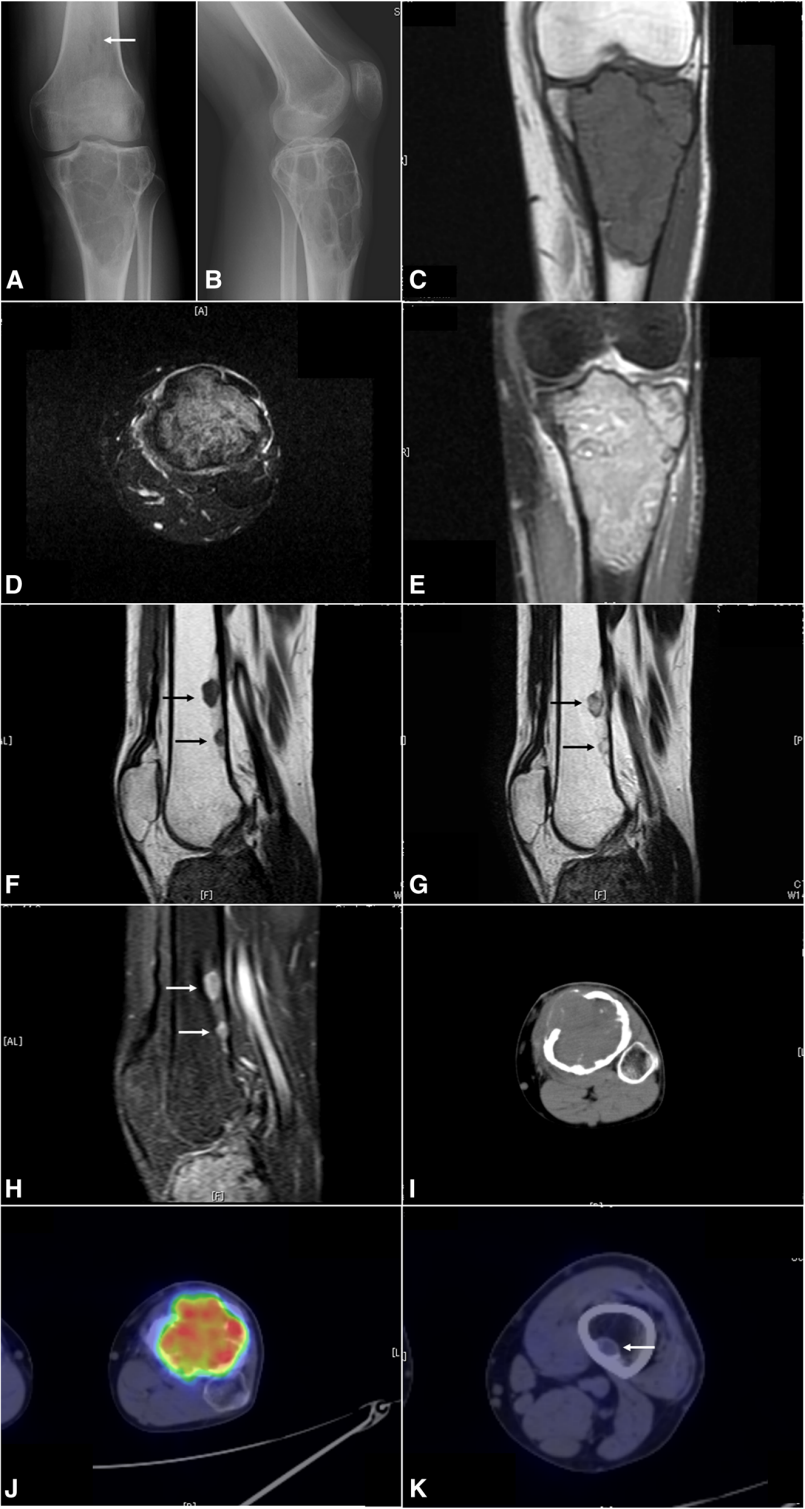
Pre-treatment radiological analyses of the left knee of the patient. Plain radiographs show a soap-bubbly osteolytic lesion with thinned cortical bone in the epiphysis of the proximal tibia (A, B) and small osteolytic lesions with a nonsclerotic margin in the metaphysis of the distal femur (arrow). MRI shows a proximal tibial tumor displaying iso-intensity to the surrounding muscle on T1-weighted imaging (coronal view) (C), heterogeneous high intensity on T2-weighted fat-suppression imaging (axial view) (D), and diffuse enhancement on gadolinium-enhanced T1-weighted fat-suppression imaging (coronal view) (E). Enhancement of surrounding soft tissue which indicates an occult pathological fracture is also observed. Sagittal MRI of the distal tibia shows small lesions (arrows) displaying nearly the same patterns as the tibial tumor; iso-intensity to the surrounding muscle on T1-weighted imaging (F), high intensity on T2-weighted imaging (G), and diffuse enhancement on gadolinium-enhanced T1-weighted fat-suppression imaging (H). ^18^F-FDG PET/CT revealed the proximal tibial tumor showing marked bone destruction (I) with increased SUV uptake (SUVmax: 9.6) (J) and the distal femoral lesions with slightly increased SUV uptake (SUVmax: 0.7) (arrow) (K).
